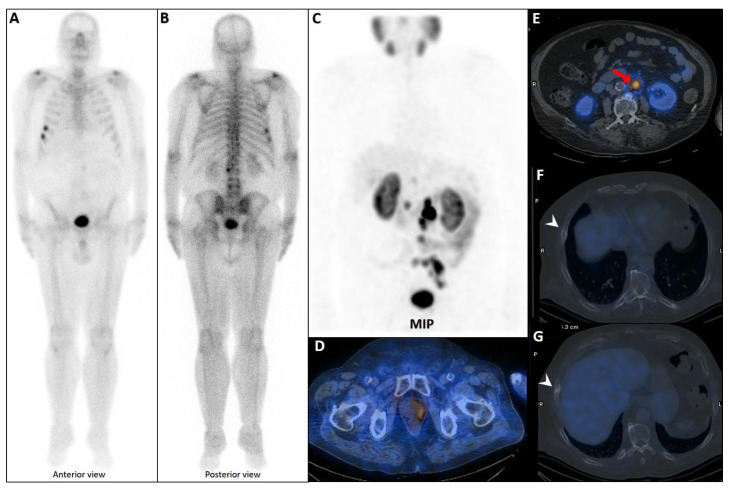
False positive findings in bone scintigraphy due to rib fractures. A 78-year-old man with prostate adenocarcinoma (biopsy Gleason score 4 + 4, serum PSA = 56.8 ng/mL) was referred for staging. Whole-body bone scan revealed two consecutive focal osteoblastic activities in the lateral arc of the ribs on the right side (A,B). 99mTc-PSMA SPECT/CT (C) revealed increased tracer uptake in the prostate gland (D), multiple metastatic lymphadenopahies on the left side of pelvis and para-aortic regions (red arrow, (E)). Moreover, fractures in the right 6th and 7th ribs showed no PSMA avidity (white arrowheads, (F,G)).
